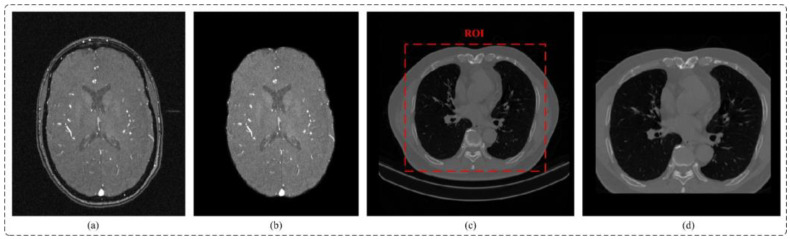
(a) TOF-MRA image (b) TOF-MRA after skull removal (c) CT image (d) Reduction of non-parenchymal regions.
